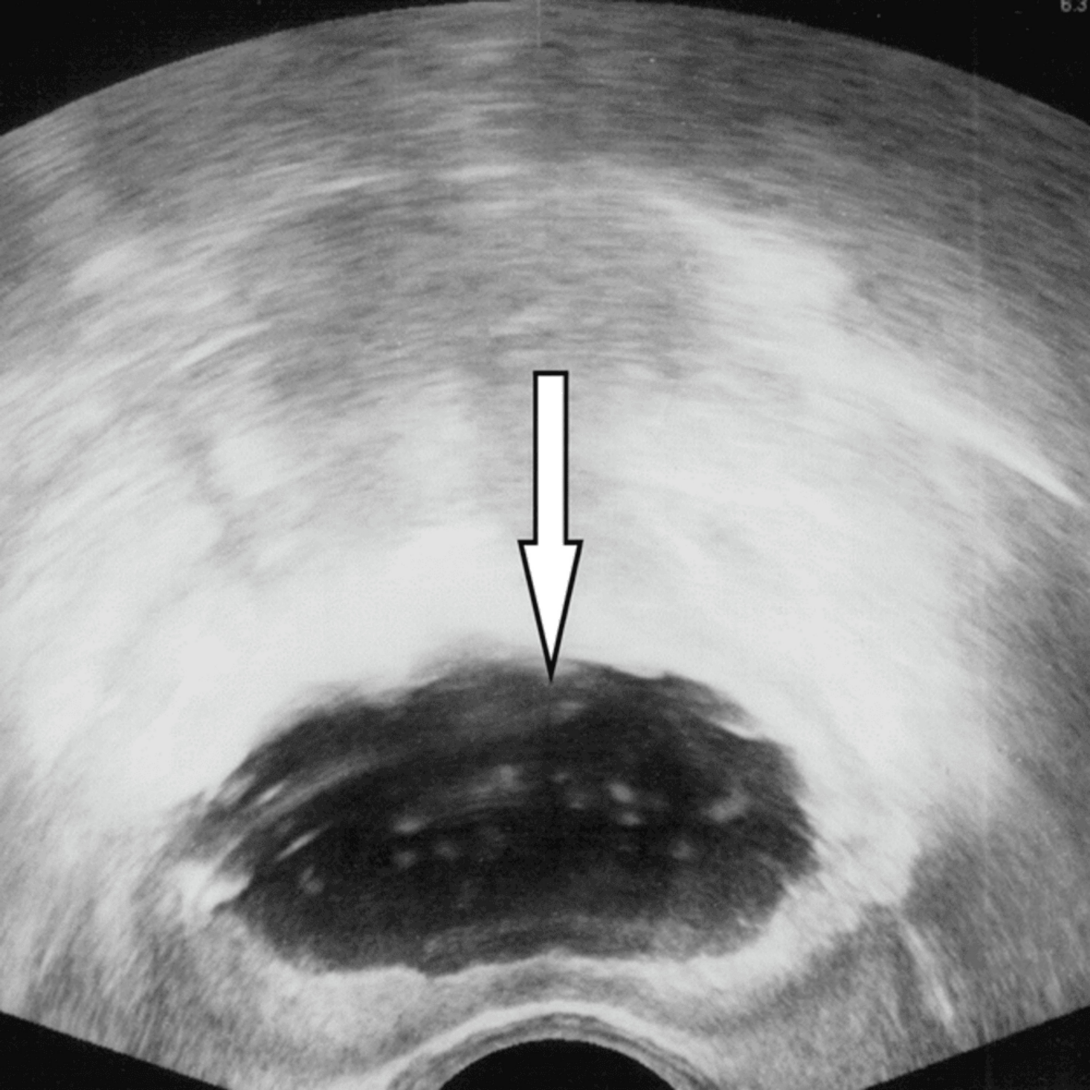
TRUS after targeted clearance complete showing resolution of shadowing and a smooth, symmetric channel with digital arrow marking a smooth symmetric channel (original image). Source: Authors; no copyrighted material used; permission not required. TRUS: Transrectal ultrasound
